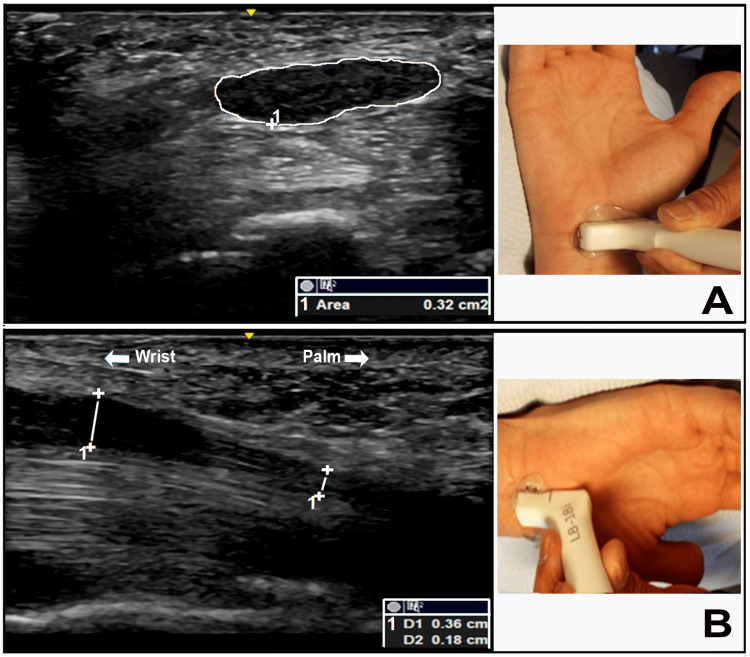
Ultrasound of the median nerve at the carpal tunnel inlet and wrist (A) Ultrasound short axis view of the right median nerve at the carpal tunnel inlet showing a significant increase in cross-sectional area (0.32 cm^2^) compared to the upper limit of normal (0.12 cm^2^). (B) Ultrasound long axis view of the right median nerve at the wrist showing a drop in diameter (left vertical line: 0.36 cm, right vertical line: 0.18 cm) within the carpal tunnel.
